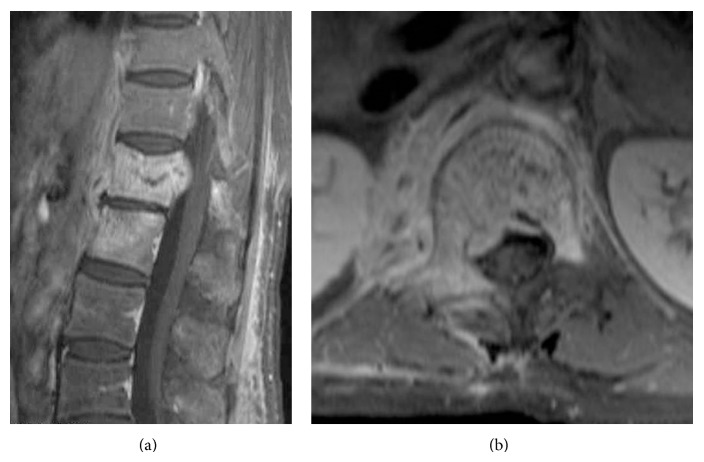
Enhanced T1-weighted magnetic resonance imaging showing vertebral osteomyelitis which involved the L1 and L2 vertebral bodies. (a) Sagittal view. (b) Axial view.
